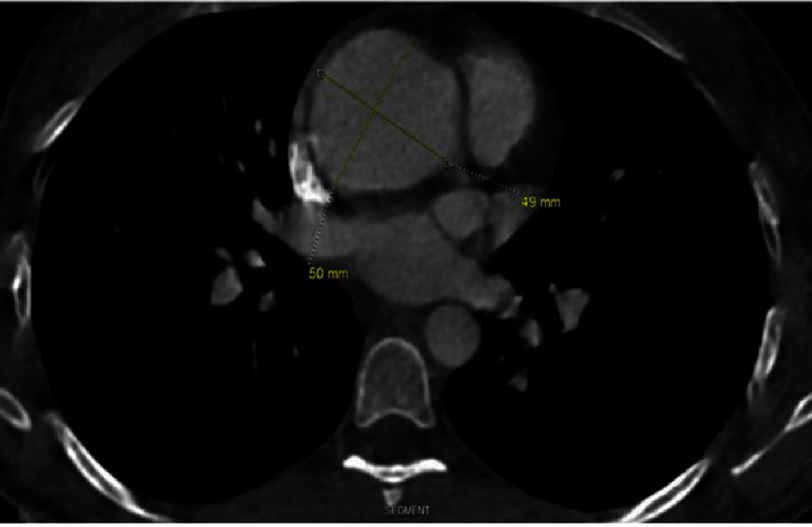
CT angiography of the chest demonstrating a 5.0 cm proximal aortic aneurysm.
